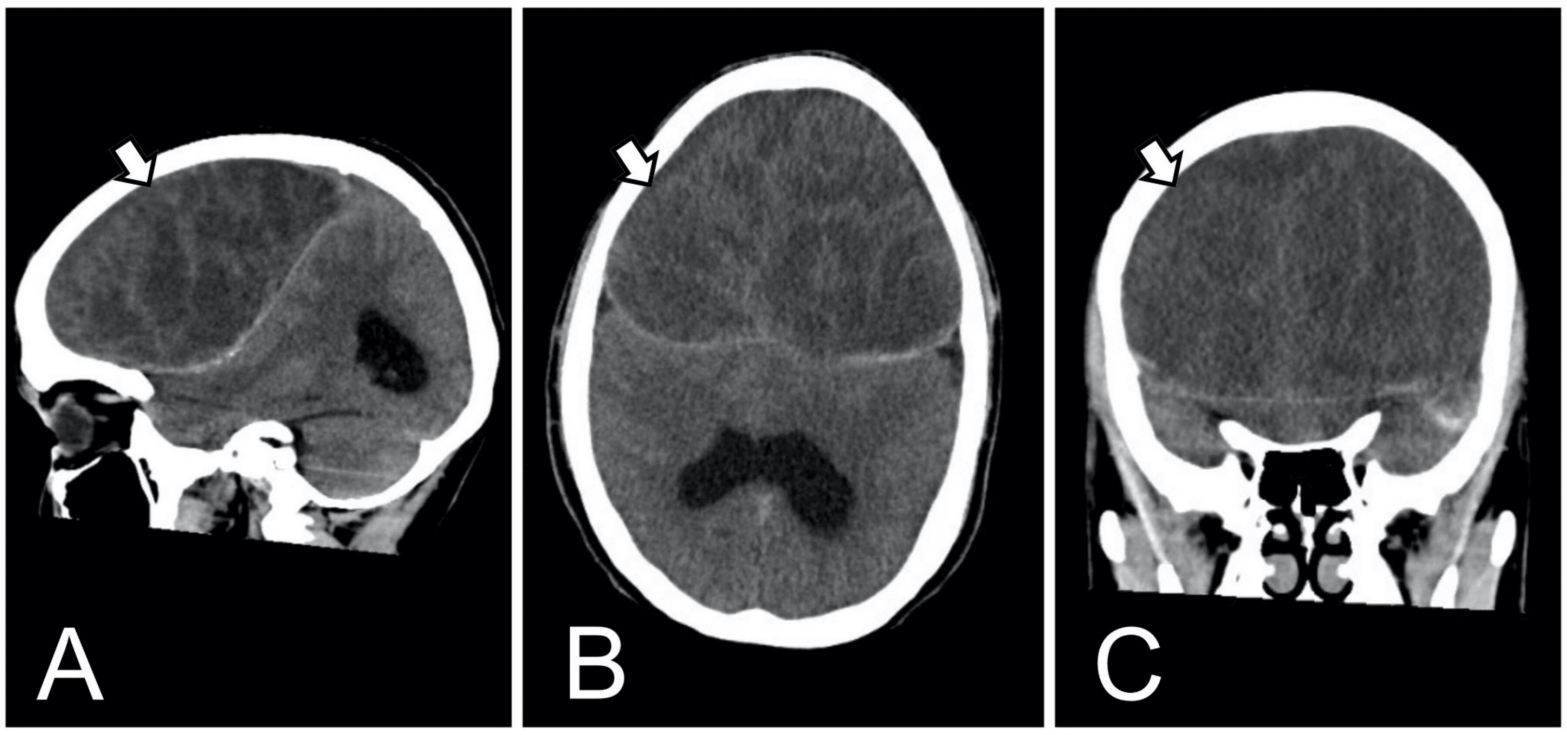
Non-contrast cranial computed tomography (CT) CT scans with sagittal (A), axial (B), and coronal (C) views. Revealed heterogeneous lesions in the frontal region. These findings were suggestive of chronic membranes with evidence of rebleeding and a mass effect.
